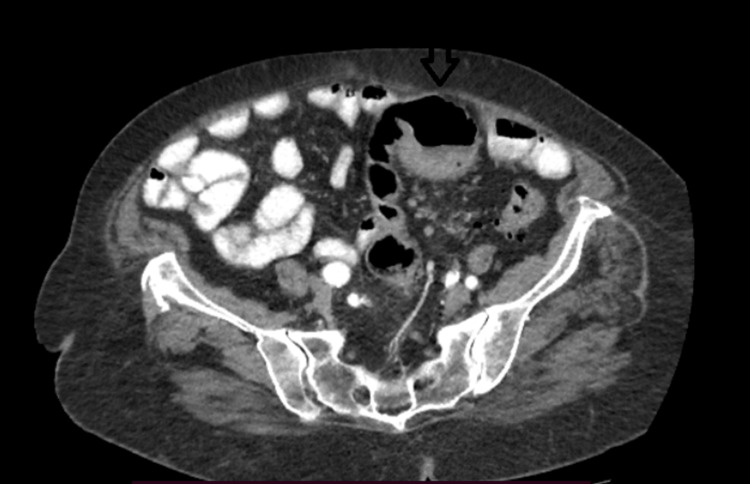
CT scan revealing distended colonic (sigmoid) loops
